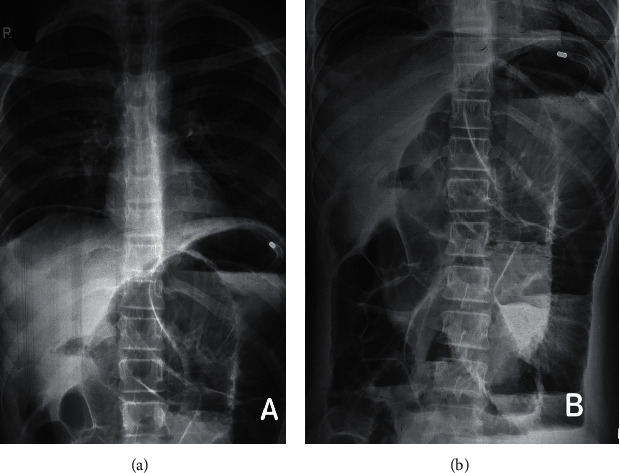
Free subdiaphragmatic extr luminal air and distended small bowel shadow in erect chest X-ray (a) and supine abdominal X-ray (b).
